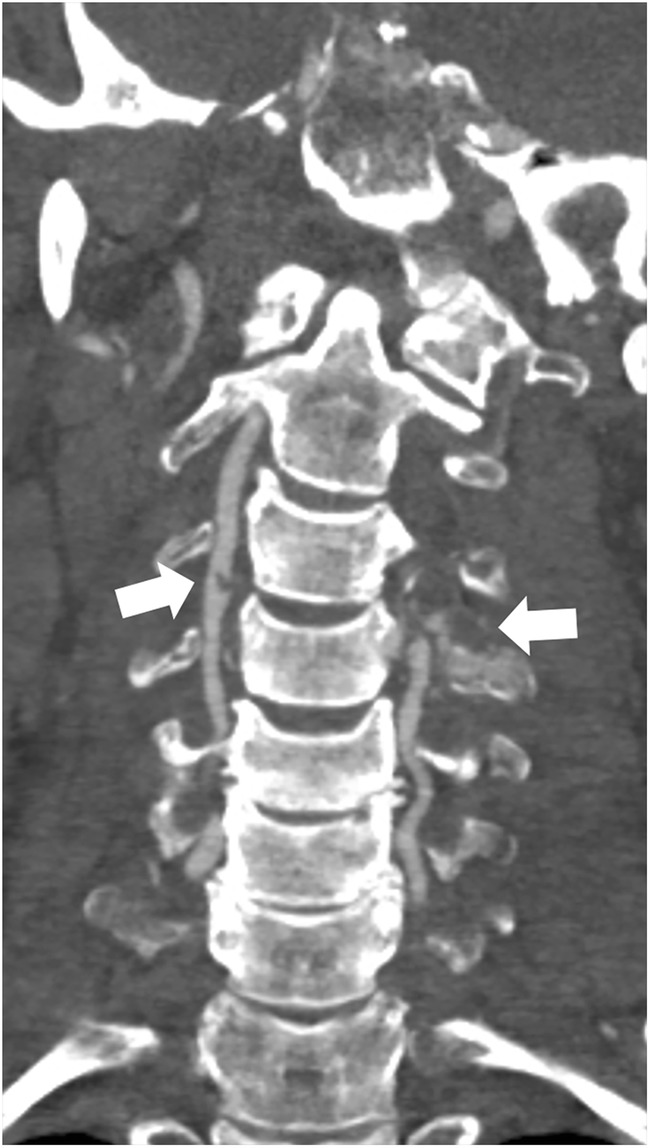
Grade II BCVI of the right vertebral artery and grade 5 BCVI of the left vertebral artery (arrows) on CTA in a 58-year-old male pedestrian who was hit by a car at 40 km/h
